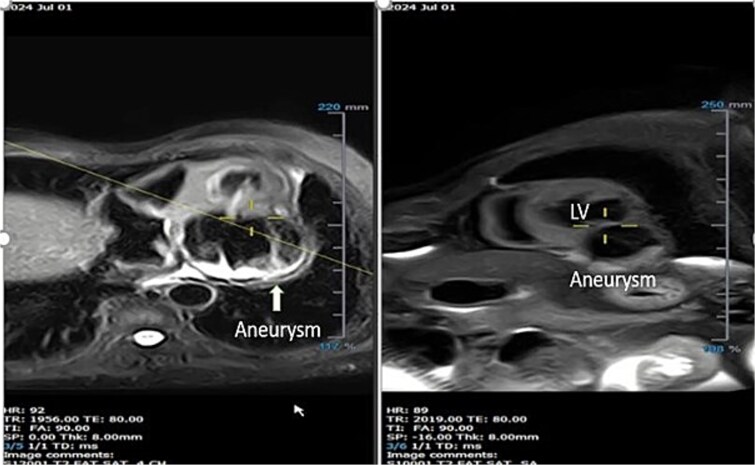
T2-weighted imaging stills focusing on the LV aneurysm in long-axis (a) and short-axis (b) views, showing diffuse hyperintense signals throughout the aneurysmal wall.
